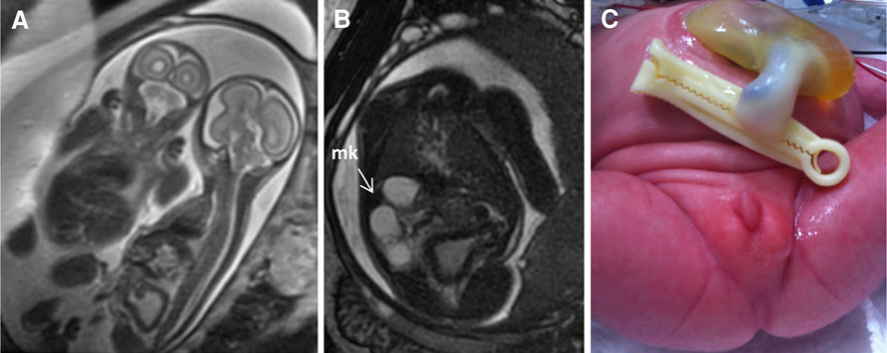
Fetal MRI of monochorionic, monoamniotic twins showing affected twin on the *right* with distended thick bladder (a, b), multicystic kidney = mk (b), and postnatal picture showing imperforate anus and umbilical cord cyst (c)
